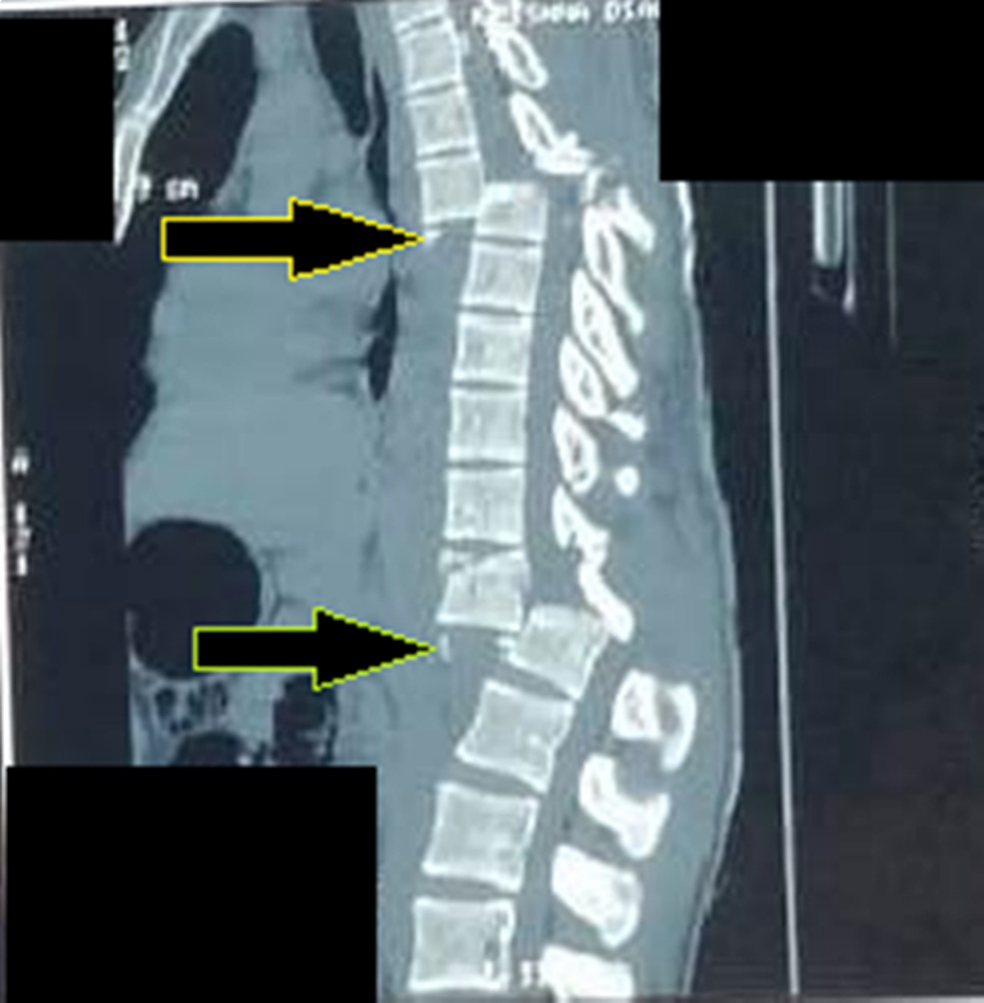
Preoperative mid-sagittal section of CT demonstrates the extent of translation at T6-T7 (proximal arrow) and T12-L1 (distal arrow)
